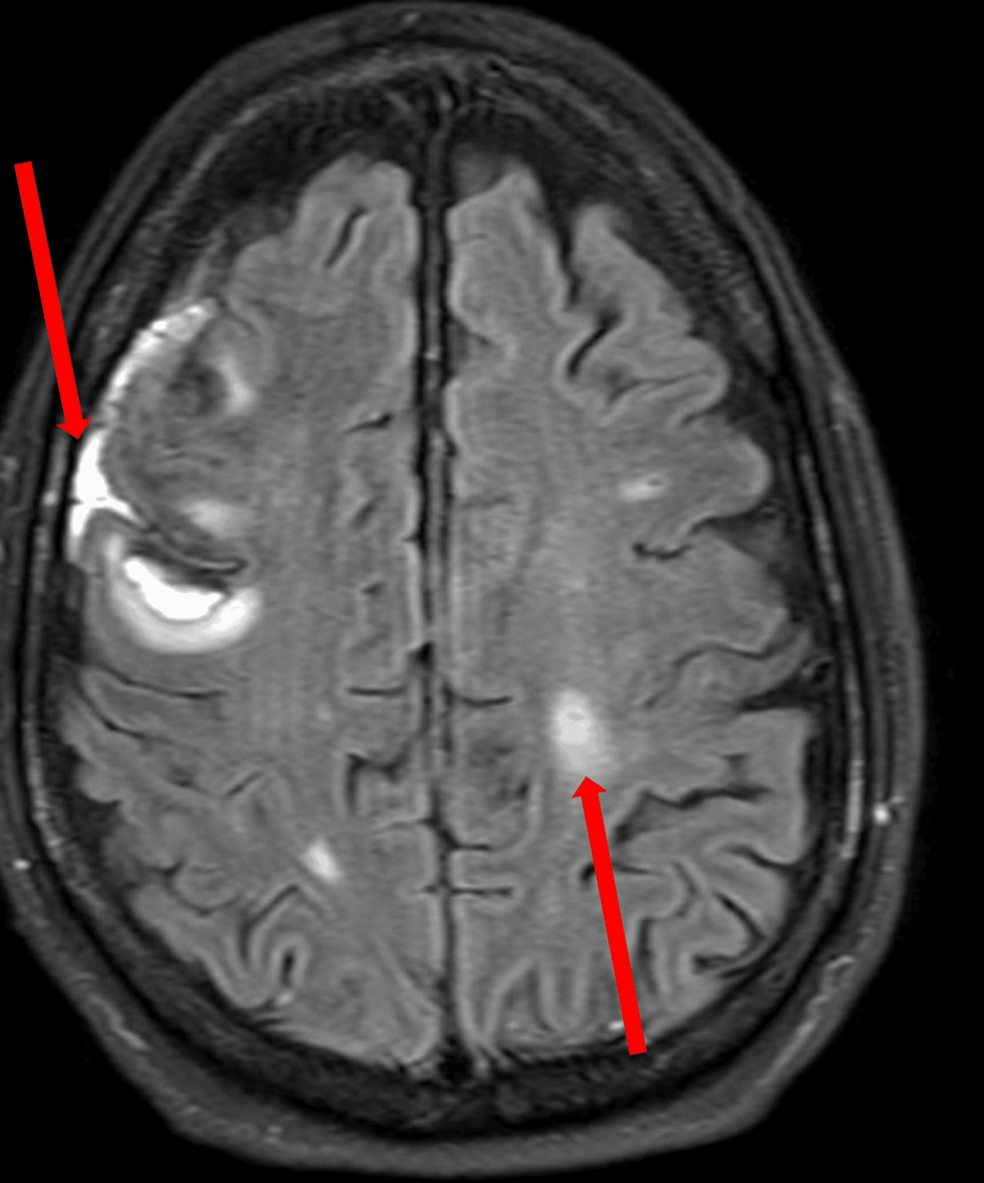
The FLAIR imaging shows evolving extra-axial and parenchymal hemorrhages with areas of cerebritis; phlegmonous changes are visualized on the right (arrows). FLAIR: fluid-attenuated inversion recovery
